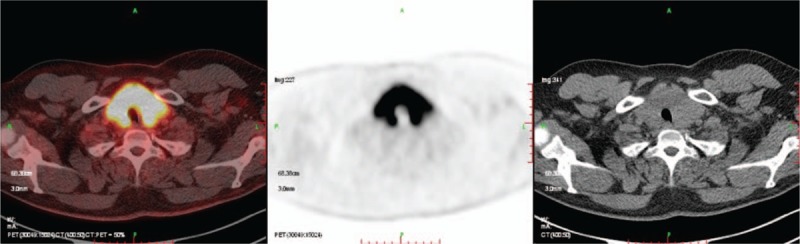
Positron emission tomography/computer tomography (PET/CT) showed extensively abnormal hypermetabolism in the thyroid. The unusual lesion might be caused by Langerhans cell histiocytosis; however, papillary thyroid carcinoma could not be excluded.
